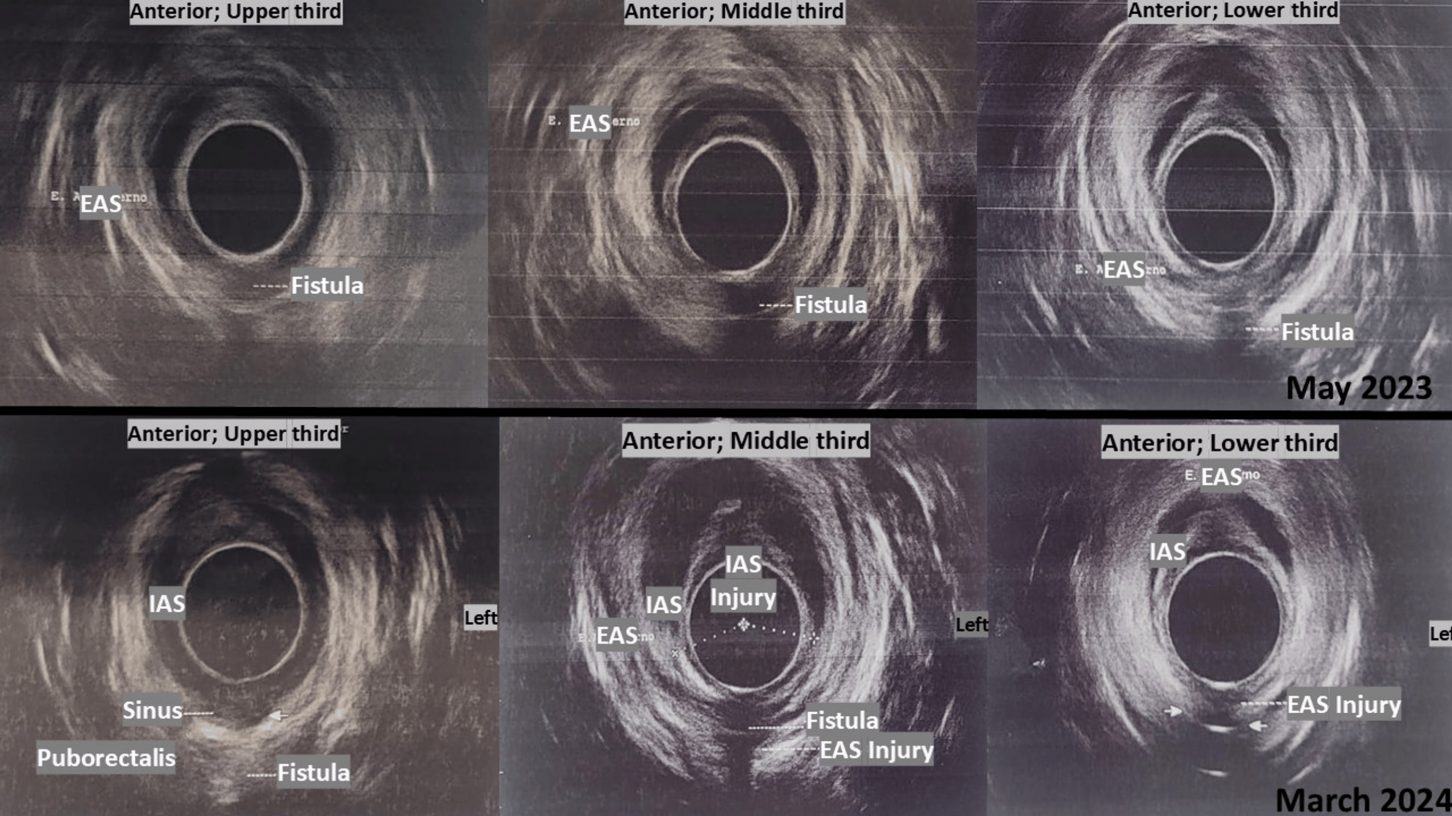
Endoanal ultrasound findings (May 2023 and March 2024) 360° endoanal ultrasound images showing the progression of a complex posterior transsphincteric fistula. In May 2023 (top row), a hypoechoic fistulous tract is visualized. In March 2024 (bottom row), recurrence with a fibrotic sinus tract extending toward the puborectalis muscle is observed, along with clear disruption of both the internal and external anal sphincters. EAS, external anal sphincter; IAS, internal anal sphincter.
